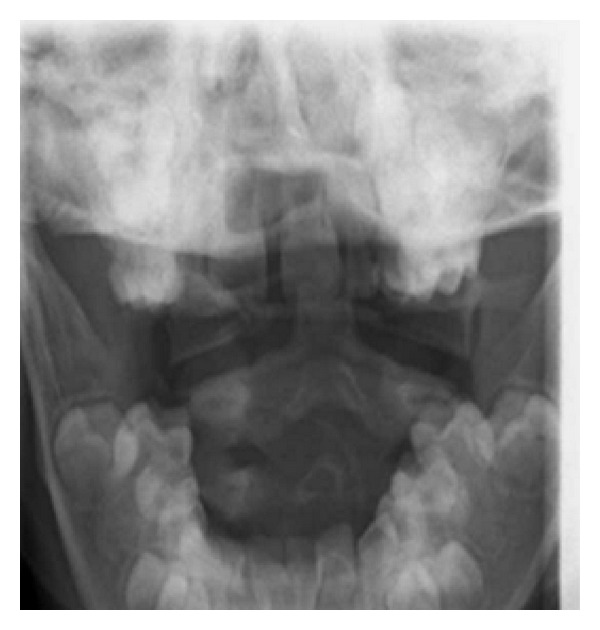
Open-mouth X-ray of the odontoid peg suggesting transverse fracture with lateral displacement through the odontoid peg.
